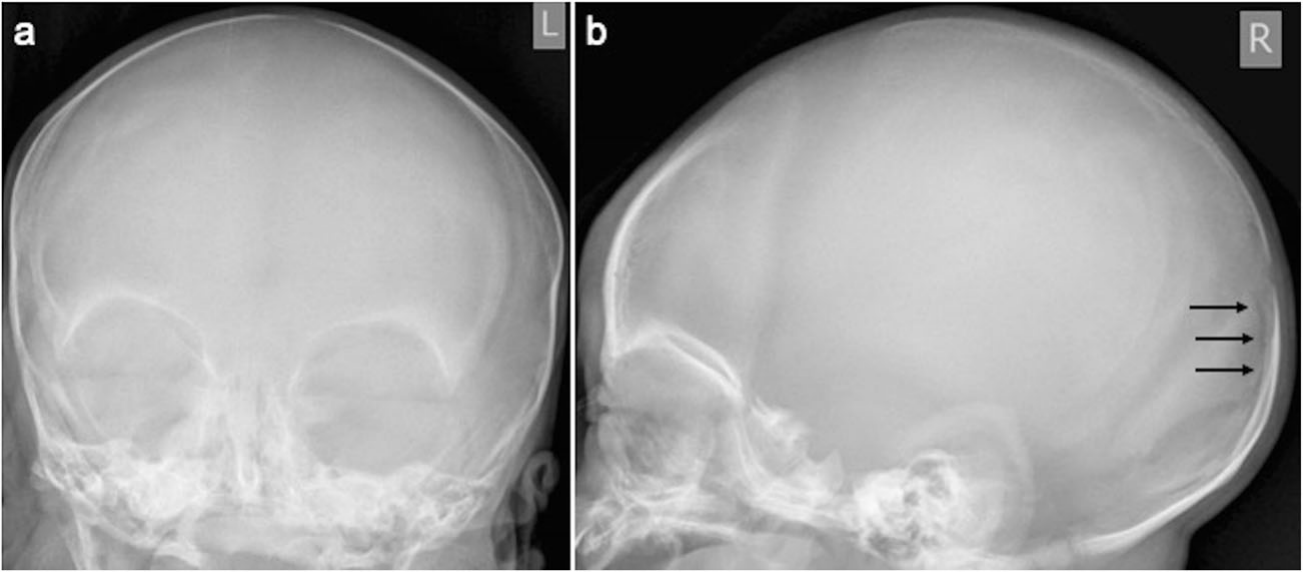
False-positive fracture on radiography: AP (a) and lateral skull (b) radiographs of a 24-day-old infant. There is a linear lucency in the occipital bone (black arrows) which was reported as a fracture by one of the reviewers
